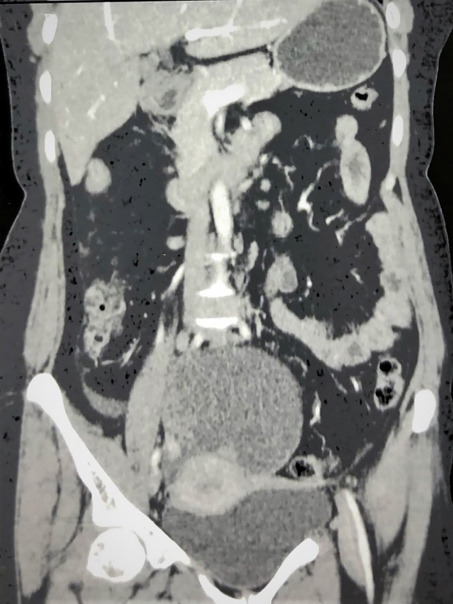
Magnetic resonance imaging (T1 weighted) shows a solid cystic ovarian mass with internal septations.
